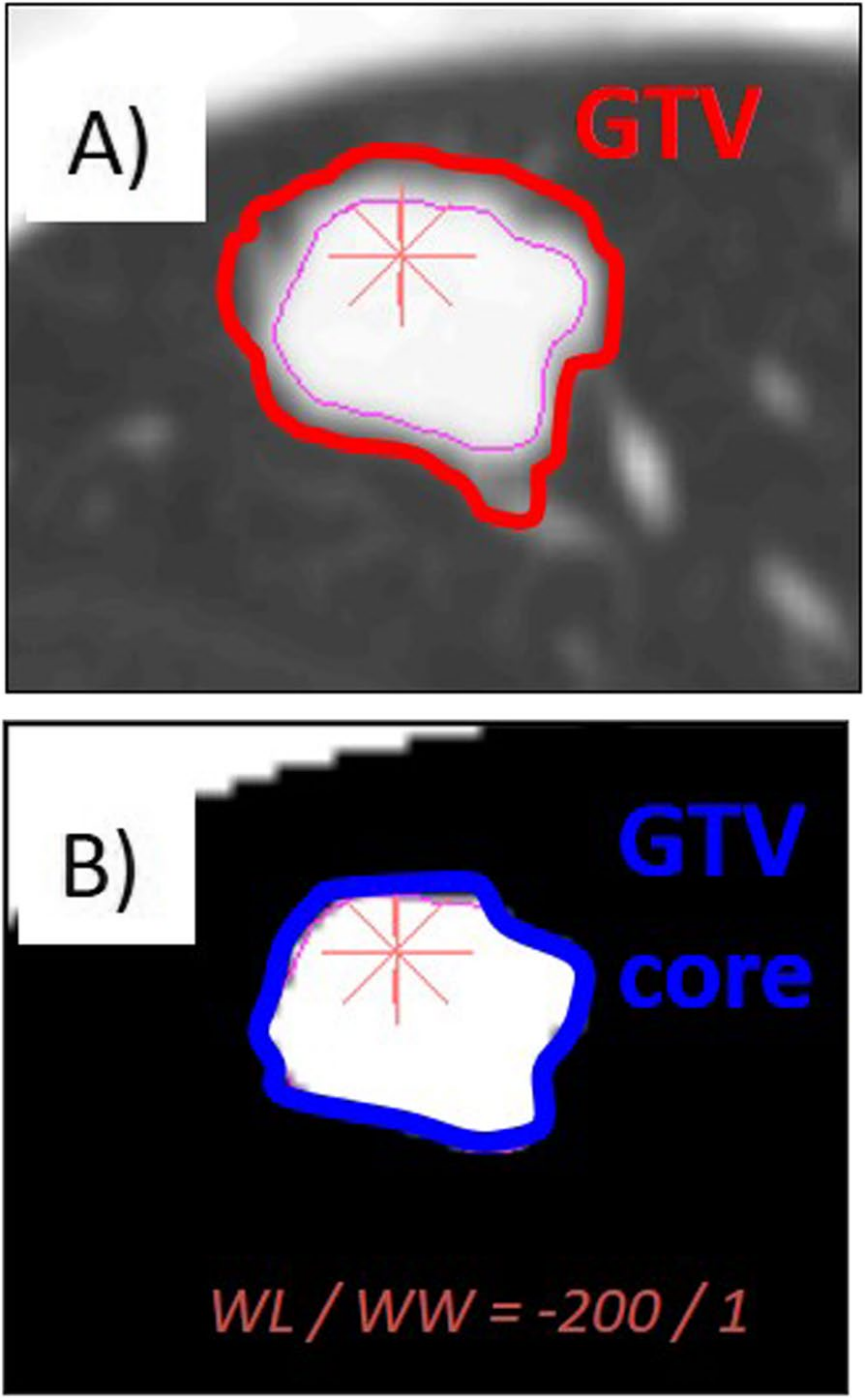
CT images of contoured GTV (A) and GTV core (the solid tumor components of the GTV) B. GTV was delineated in the lung window CT setting, while GTV core were contoured (window level [WL], –200 Hounsfield units; window width [WW], 1 Hounsfield unit). GTV, gross tumor volume
